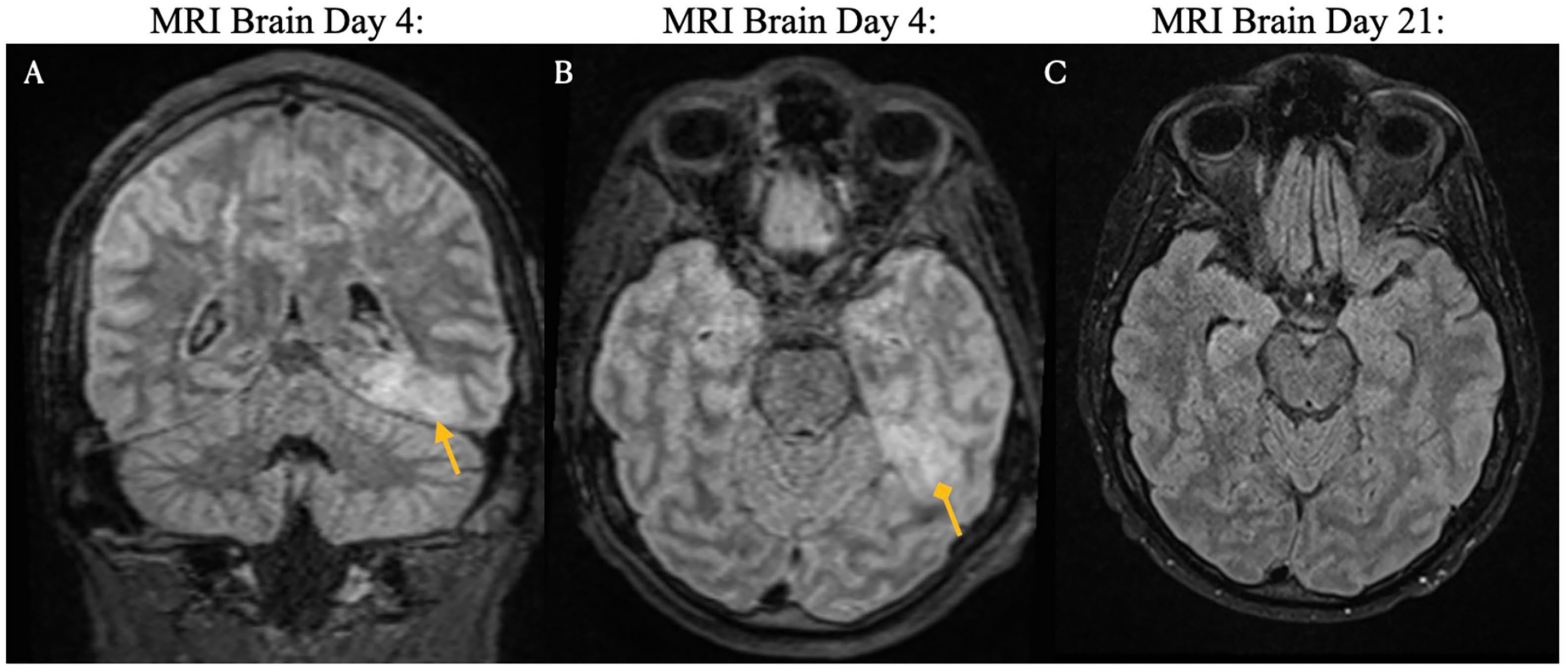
Brain magnetic resonance imaging on days four and 21 post-CAR T-cell infusion. ICANS-associated brain MRI findings showing focal FLAIR hyperintensity in the left mesial temporal lobe (Panels A,B), which may reflect recent seizure activity. Images from pre-treatment (Day 4: Panels A,B) and post-treatment (Day 21: Panel C) with steroids and Anakinra demonstrate resolution of the lesion.
